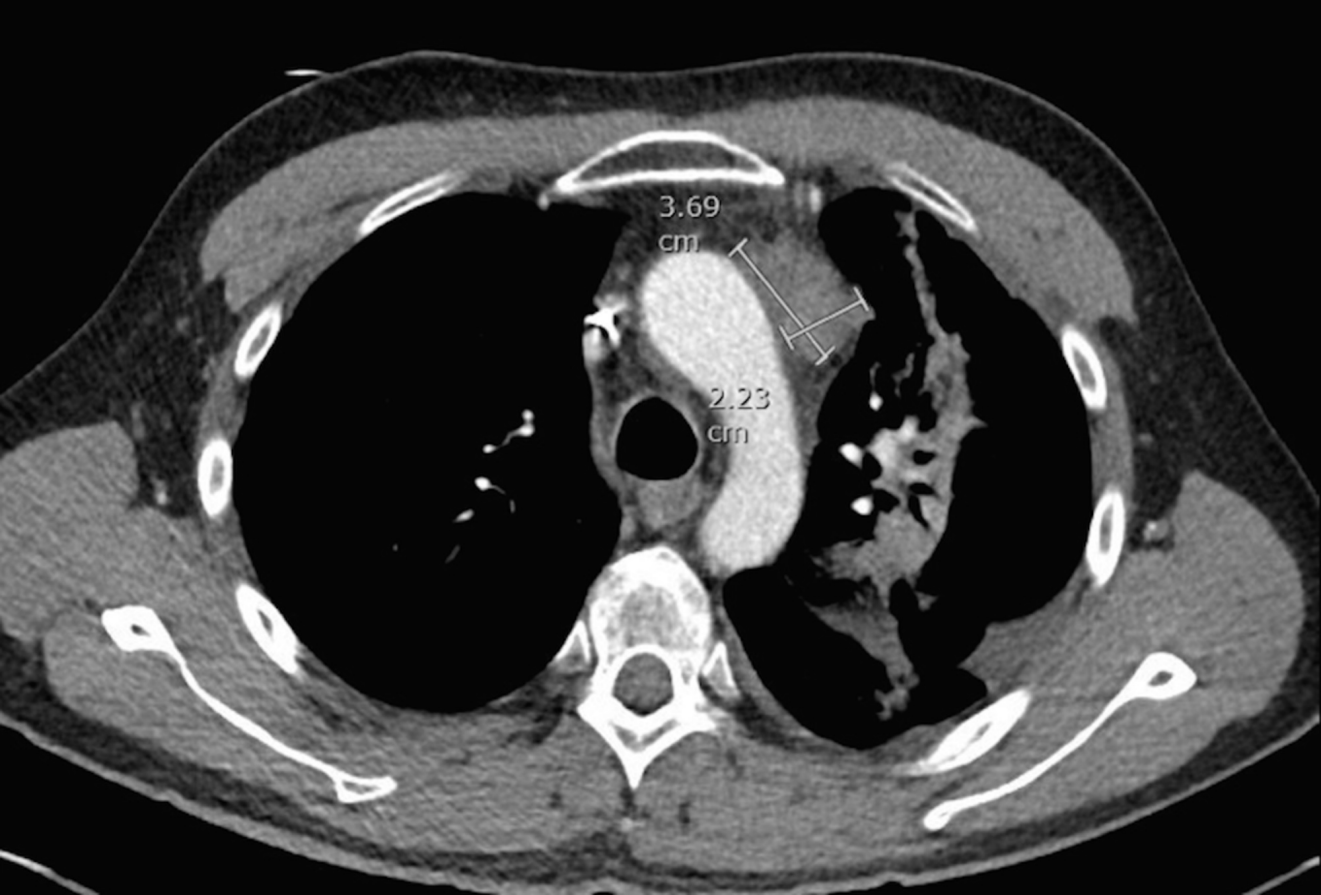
Computed tomography (CT) scan of the chest showing the anterior mediastinal mass
